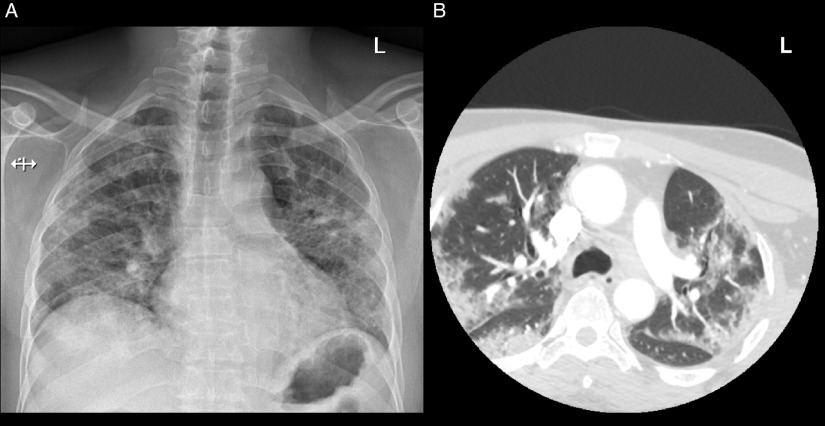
Lung imaging. (A) Chest x-ray demonstrated diffuse heterogeneous infiltration in both lungs and corresponded with (B) CTA of the head and neck, which demonstrated ground-glass opacities in the lung apices, involving primarily the periphery in the upper lobes of the lungs and the superior segments of the lower lobes. These findings were consistent with COVID-19. L = left.
